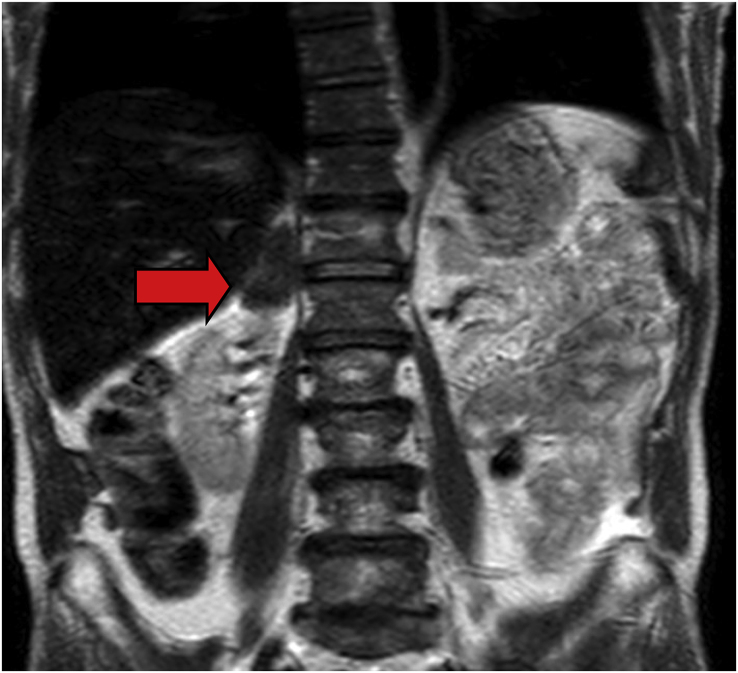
(Case 2) Day +6 Abdominal MRI: 33 mm diameter right adrenal focal lesion, which shows intermediate signal intensity at T2 and T1. The lesion is not associated with intra- or extra-cellular "fat" and presents restricted diffusion. (Red Arrow). (For interpretation of the references to colour in this figure legend, the reader is referred to the Web version of this article.)
